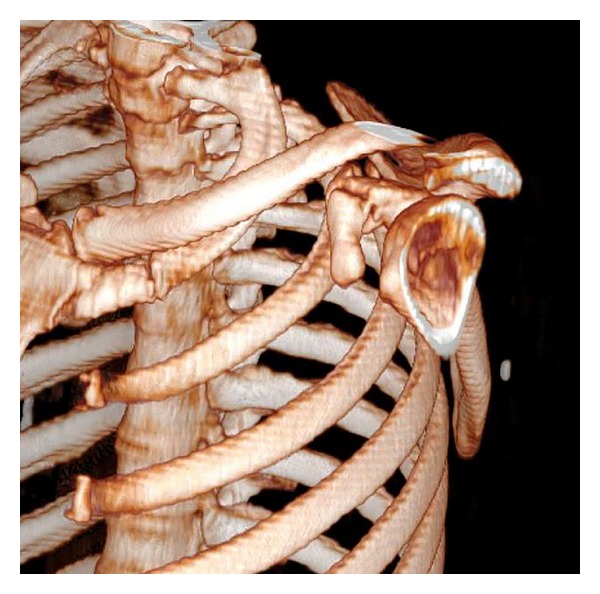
Preoperative 3-dimensional CT image.
